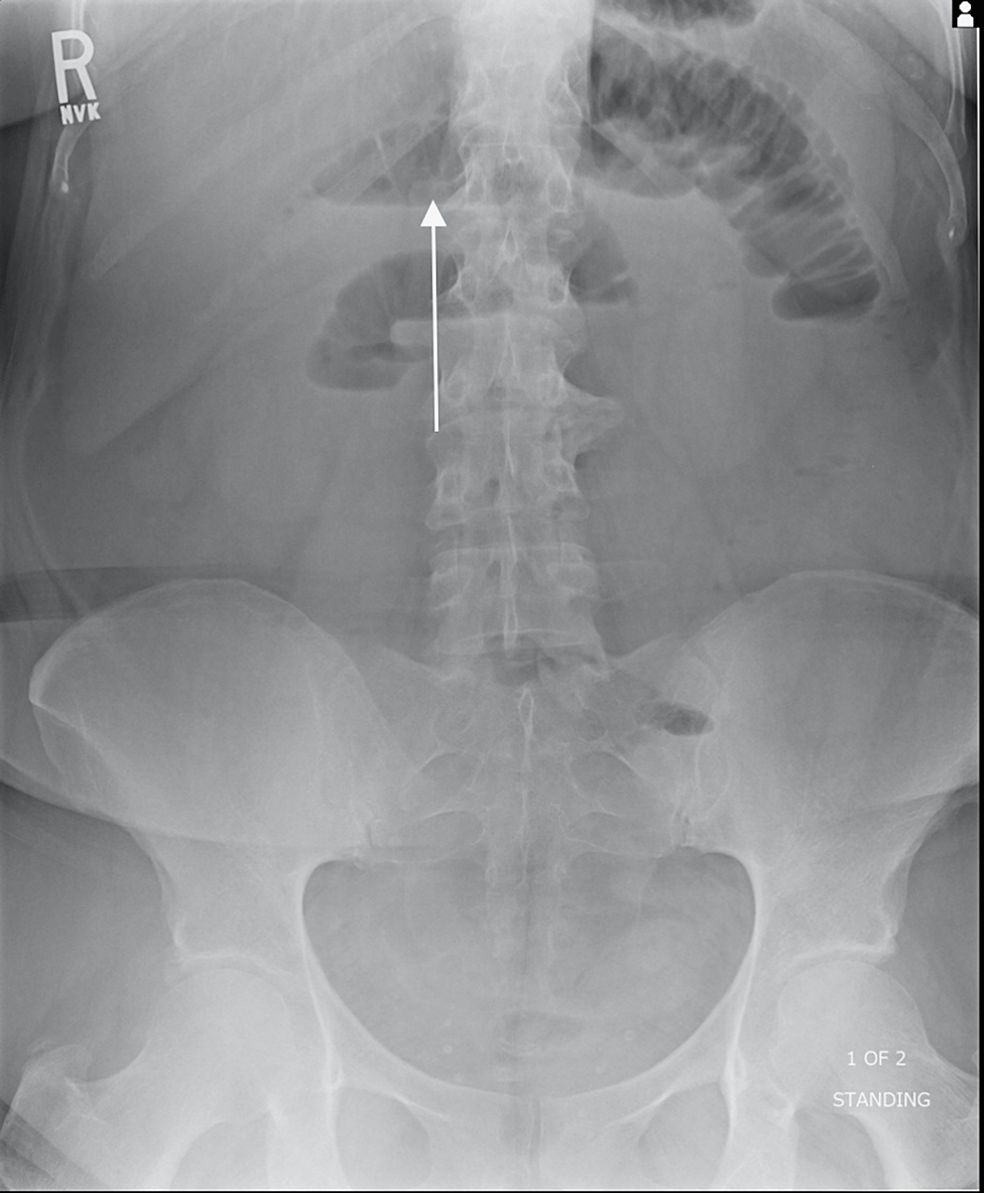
Abdominal radiograph of the upright frontal view of the abdomen demonstrating dilated loops of small bowel with air-fluid levels (arrow) and absence of gas in the colon.
